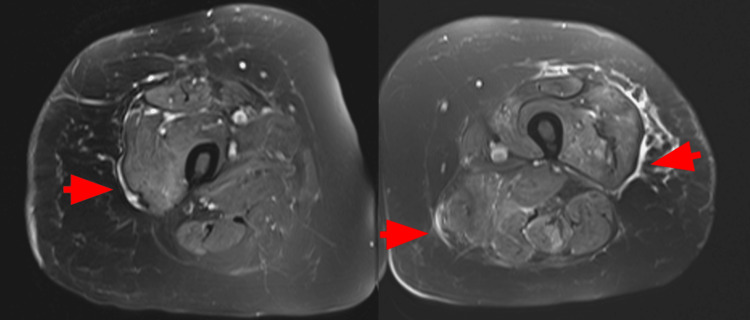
An axial T2-weighted fat sequence MRI image of bilateral femurs shows diffuse muscle edema (red arrow).
